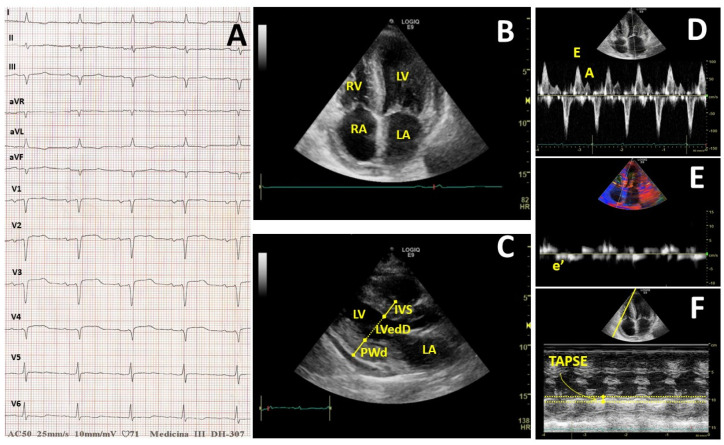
Heart involvement due to amyloid deposition will lead to different instrumental issues. One finding is low voltage diffused in all leads. (A) Other typical findings are echocardiography alterations. Increased thickness of the heart wall in bi-mode heart ultrasound both in the apical 4-chamber view (A4C) (B) and parasternal long-axis view (PLAx); (C) the latter is useful to evaluate left ventricle diameter (IVS, LVedD and PWd). A4C is useful to evaluate volumes; Doppler analysis was also performed in A4C: Pulsed wave Doppler on mitral valve (D) it was necessary to evaluate both E and A velocity, while tissue Doppler imaging (E) was used to measure e’ velocity; TAPSE was measured in A4C using monodimensional mode (F).
